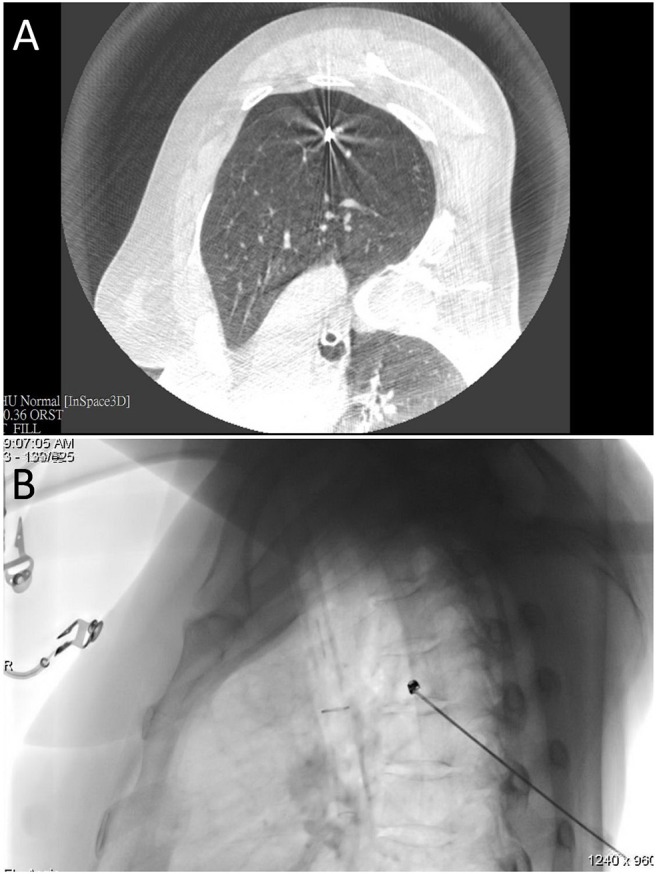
(A) An intraprocedural CT scan confirmed that the needle tip was properly positioned in the proximity of the nodule of interest. (B) Fluoroscopic view of microcoil insertion through a co-axial needle.
